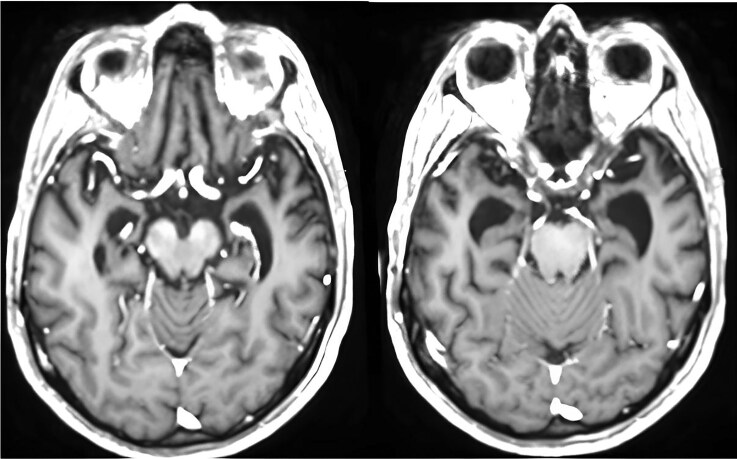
Bilateral temporal atrophy on MRI. Axial slice of T1-weighted MRI showing severe atrophy in the anterior temporal region bilaterally slightly predominating on the left (right side of the slide).
